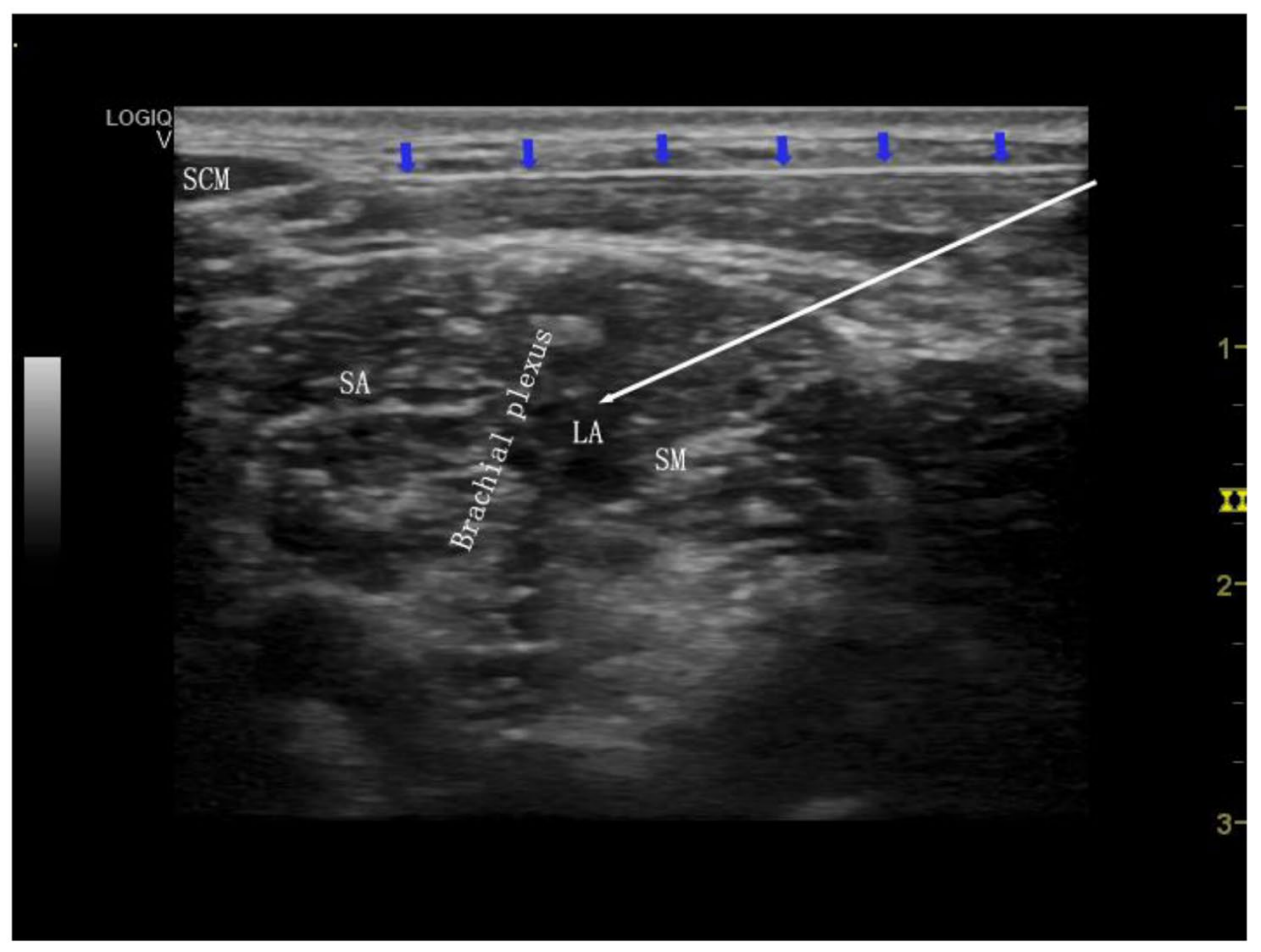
Single injection for superficial cervical fascia block combined with brachial plexus block under ultrasound guidance. SCM: Chest clavicle muscle, SA: Anterior oblique muscle; SM: Middle oblique muscle; LA: Local anaesthetic; Blue arrow: puncture needle with shallow fascia block of the neck; White arrow: needle route of brachial plexus nerve block
